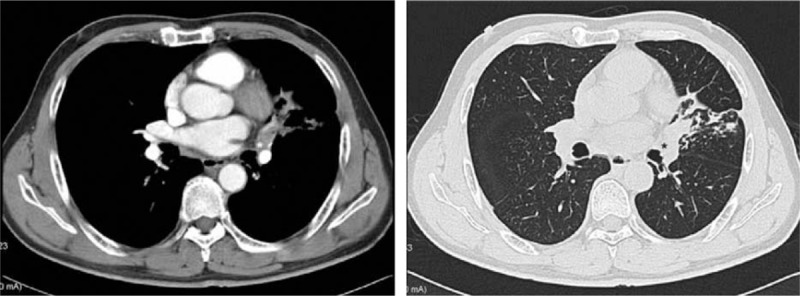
CT scan revealed a central tumor (asterisk) obstructing the left upper lobe bronchus orifice.
